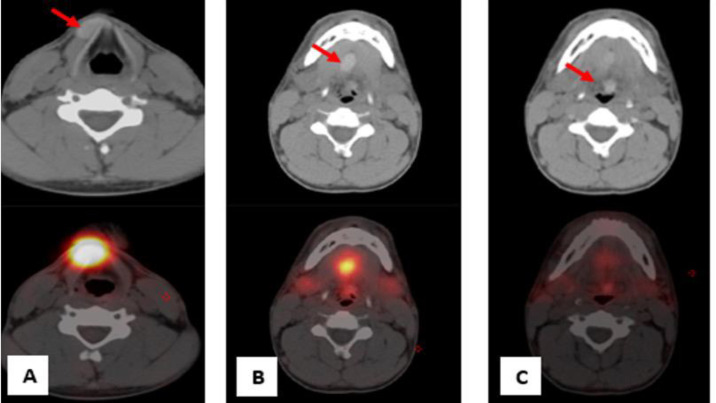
Axial non-contrast computed tomography and SPECT images showing hyperdense foci at (A) right infra-hyoid, (B) sublingual and (C) lingual regions (red arrow)
